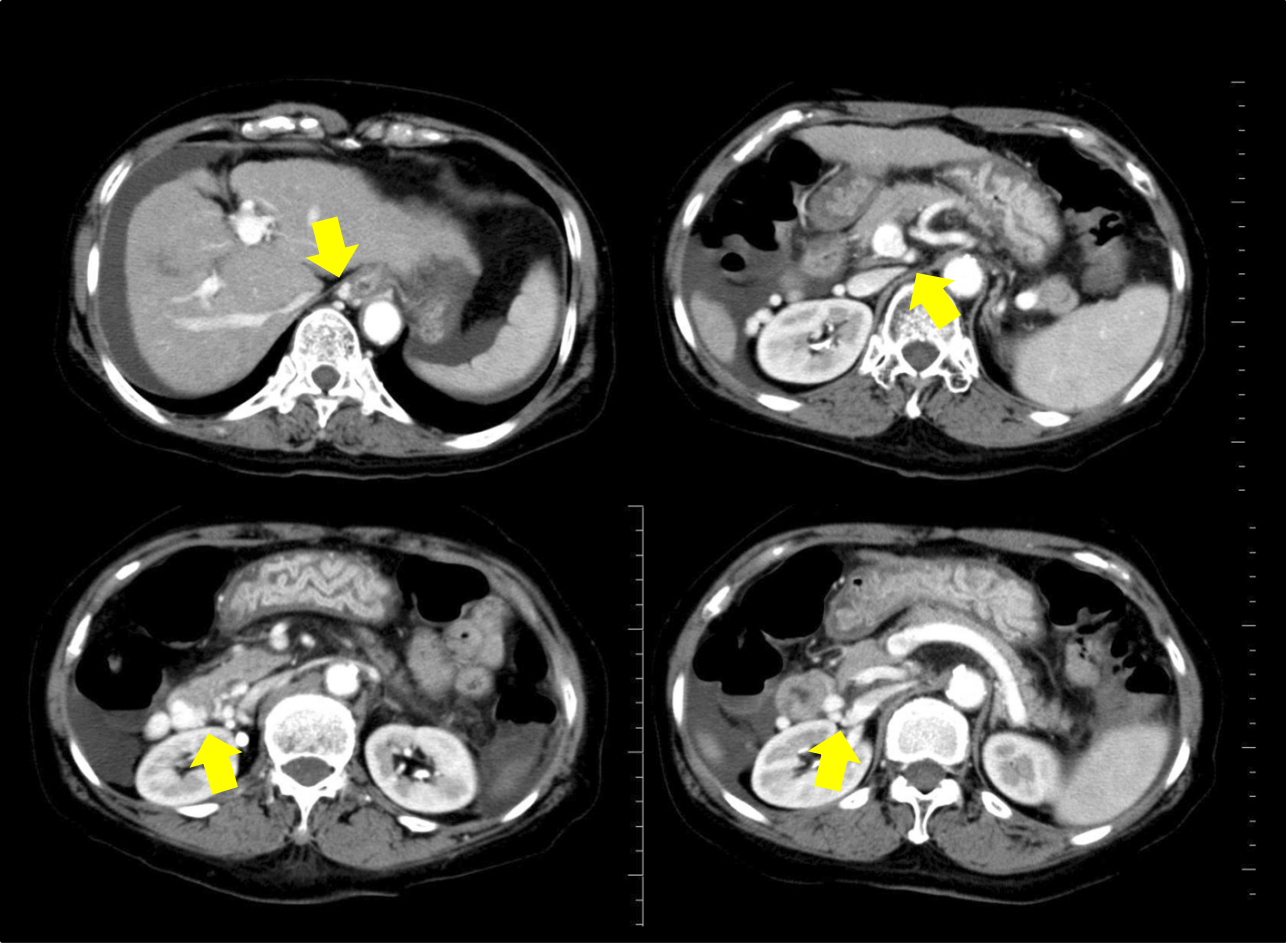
Initial abdominal computed tomographic images showing paraesophageal varices and venous collaterals around the duodenum. Extravasated contrast is seen in the second portion of the duodenum. The afferent collateral vessel originated directly from the main portal vein, and the efferent collateral vessel drained into the inferior vena cava via the ovarian veins
